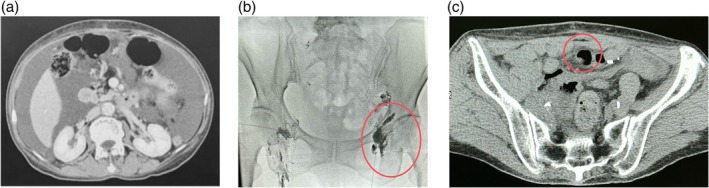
Imaging studies of chylous ascites detection and lymphatic leakage localization. (a) Abdominal CT on post‐operative day 75 showing significant accumulation of ascitic fluid in the pelvic cavity. (b) Although initial lymphangiography did not reveal any leakage, ultrasound‐guided puncture of the left inguinal lymph node with lipiodol injection confirmed intraperitoneal leakage from the left external iliac lymph node. (c) A CT scan (cross‐sectional view) after lipiodol injection into the left and right pelvic lymph nodes shows leakage of contrast medium into the abdominal cavity (leakage site marked in red), indicating lymphatic leakage originating from the left external iliac lymph nodes. The lymph fluid leakage is indicated in red.
